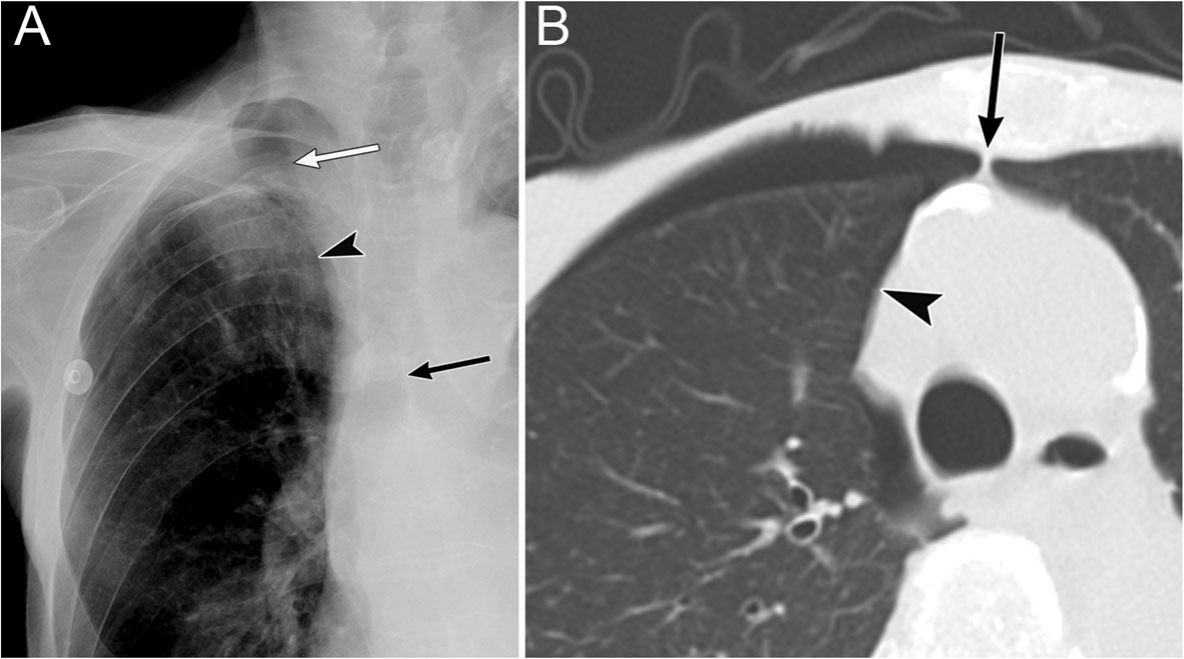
Pneumothorax in the suprahilar anteromedial space of an 83-year-old man with enteritis. a A supine radiograph shows sharp delineation of the superior vena cava (SVC) (arrowhead), right brachiocephalic vein (white arrow), and anterior junction line (black arrow). b Axial CT image at the level of the SVC shows air collection in the pleural cavity on the right sides of the SVC (arrowhead) and the anterior junction line (black arrow)
